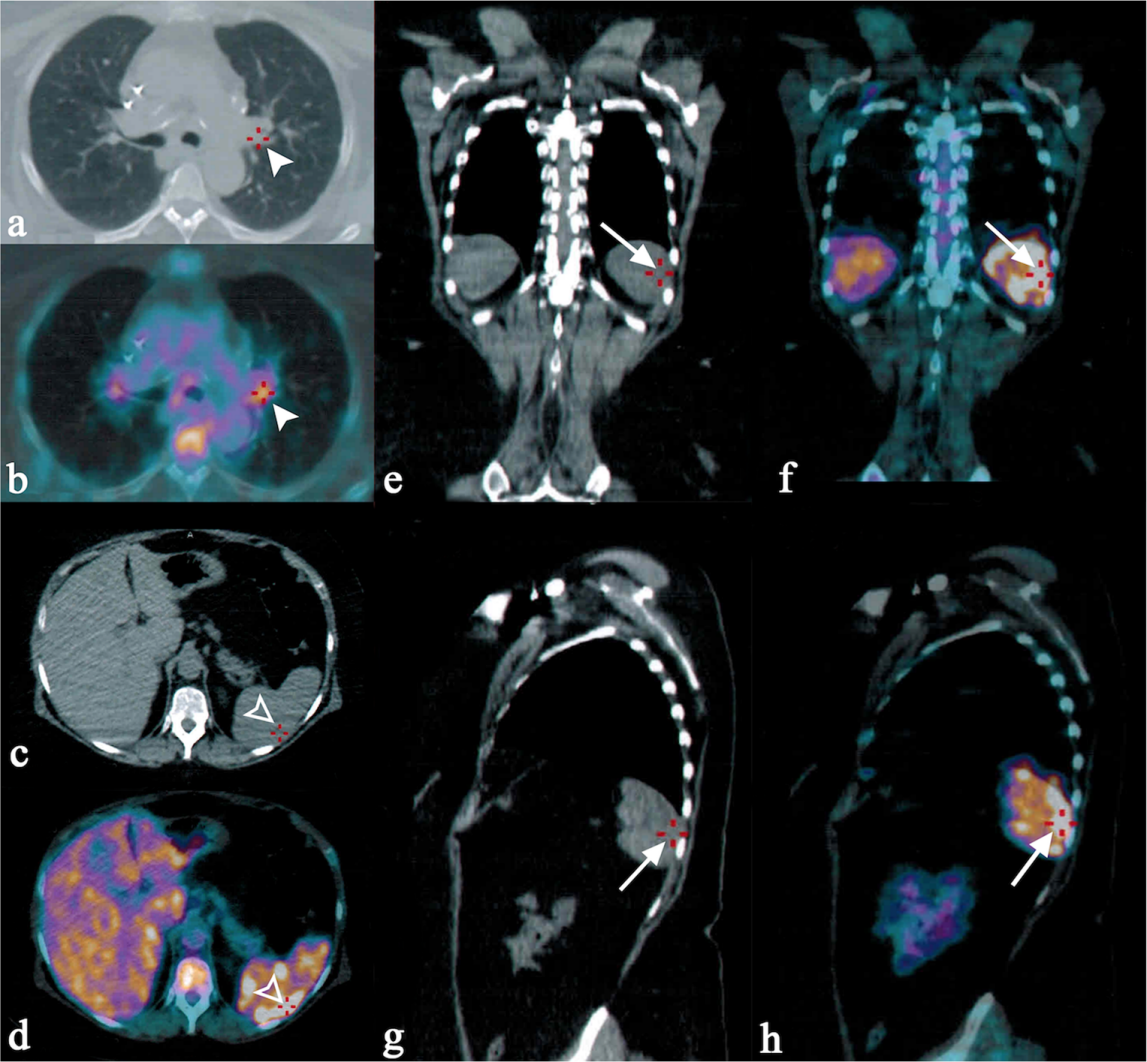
FDG uptake on PET-CT in a 57-year-old woman affected by pulmonary and splenic sarcoidosis. Increased metabolic activity is appreciable in the left pulmonary hilum, due to pathological lymph nodes (*white arrowheads* on Fig. 6a–b). Similarly, splenic foci of increased metabolic activity are clearly seen on axial CT-PET acquisitions (empty *white arrowheads* respectively on Fig. 6c–d), and on coronal and sagittal reformatted images (*white arrows* in Fig. 6e–h)
